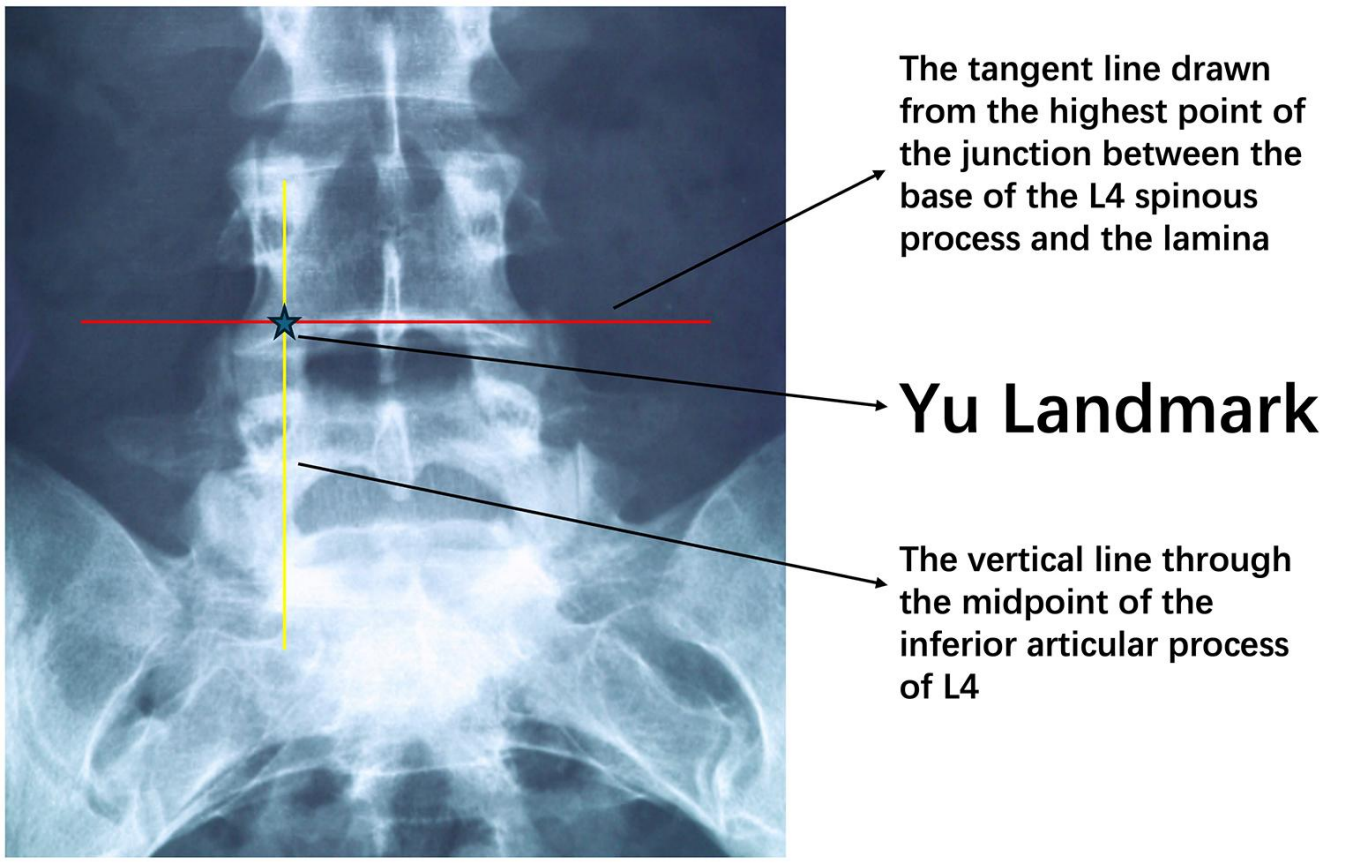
Intraoperative fluoroscopic validation of the Yu landmark concept. Anteroposterior x-ray imaging of the L4/L5 region demonstrates the same anatomical reference lines as shown in Figure 1. The vertical line passes through the midpoint of the L4 inferior articular process, and the horizontal line is tangent to the highest point of the junction between the L4 spinous process base and lamina. The convergence point of these two lines (indicated by asterisk) represents the Yu landmark, indicating the ideal puncture position. This intraoperative fluoroscopic image confirms that the Yu landmark is easily identifiable within the patient's body and provides reliable localization guidance for surgeons.
